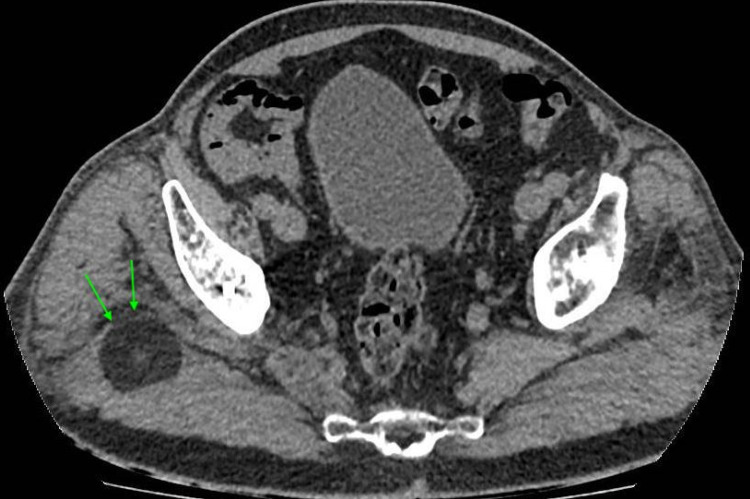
A cross-section image from a pelvic CT scan showing the dislocated polyethylene liner demonstrated by green arrows. CT: computed tomography
